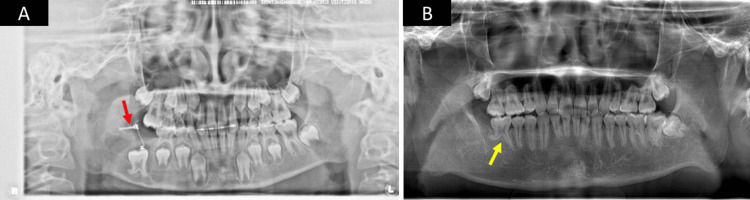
Postoperative panoramic radiograph of Case 1 (A) A panoramic radiograph obtained eight months postoperatively showing bone regeneration, an anchoring device placed at the crown of the first molar, and anchor screw implantation (red arrow) at the right mandibular area; (B) A panoramic radiograph obtained 10 years postoperatively showing regenerated bone at the right mandibular area. The first molar, which still had morphological abnormalities in the tooth root, exhibited a normal direction of eruption (yellow arrow).
